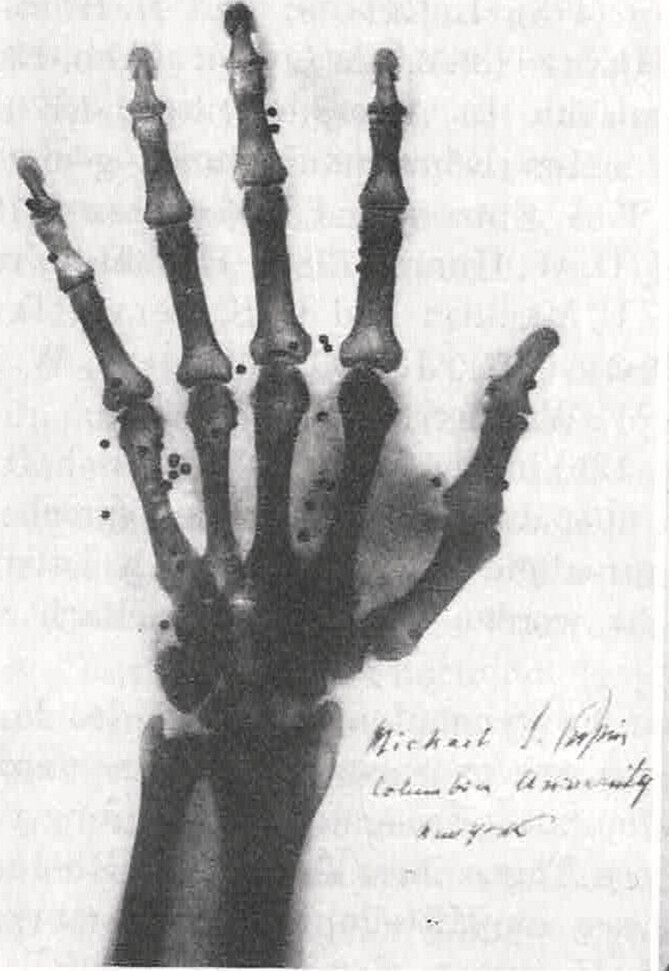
A forgery of the “hand with gunshot” X-ray published in Röntgen’s biography by Glasser in 1931. Reproduced as published. Glasser O. Wilhelm Conrad Röntgen und die Geschichte der Röntgenstrahlen. Berlin, Verlag von Julius Springer, 1931:27 [rerif]
